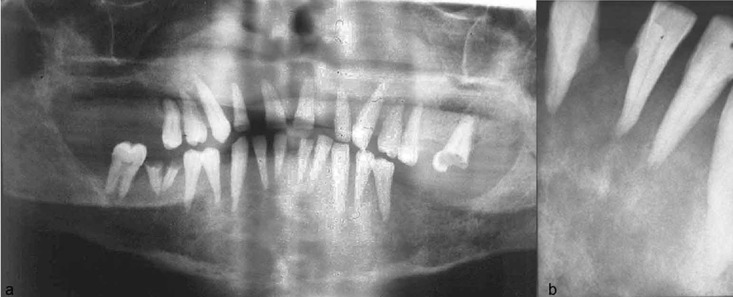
a: Panoramic radiograph showed generalized demineralization in jaw bones. The alveolar canal was unclear and the cortex was ill-defined on the right side. The posterior part of the right cortex was destroyed.  b: Lamina dura was not observed in the periapical radiograph. Severe bone loss was observed between the anterior incisors.
